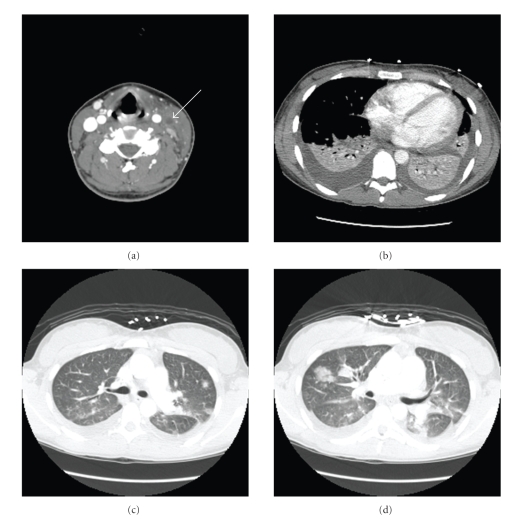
Neck CT scan (a) with contrast reveals left IJV thrombosis noted by the arrow. Chest CT scan reveals bilateral effusions (b) and multiple septic emboli (c and d).
